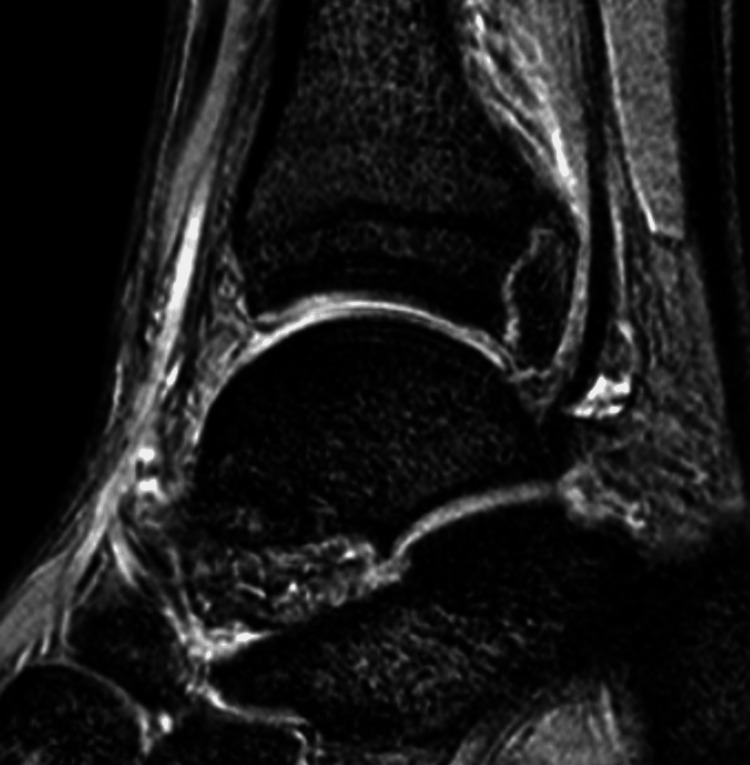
Preoperative T2-weighted sagittal magnetic resonance FHL: flexor hallucis longus Imaging shows tenosynovitis around FHL
